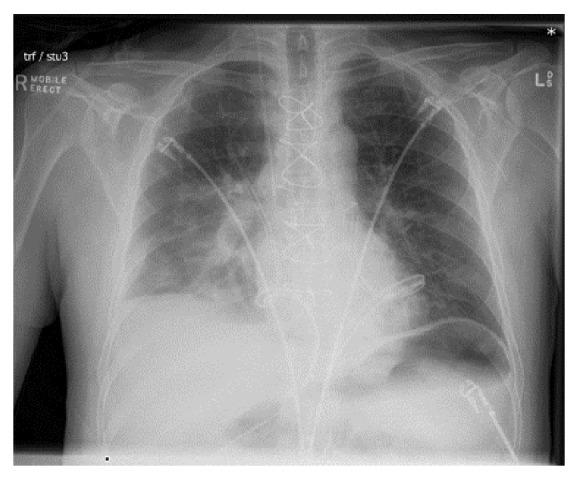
Prebronchoscopy chest X-ray.
